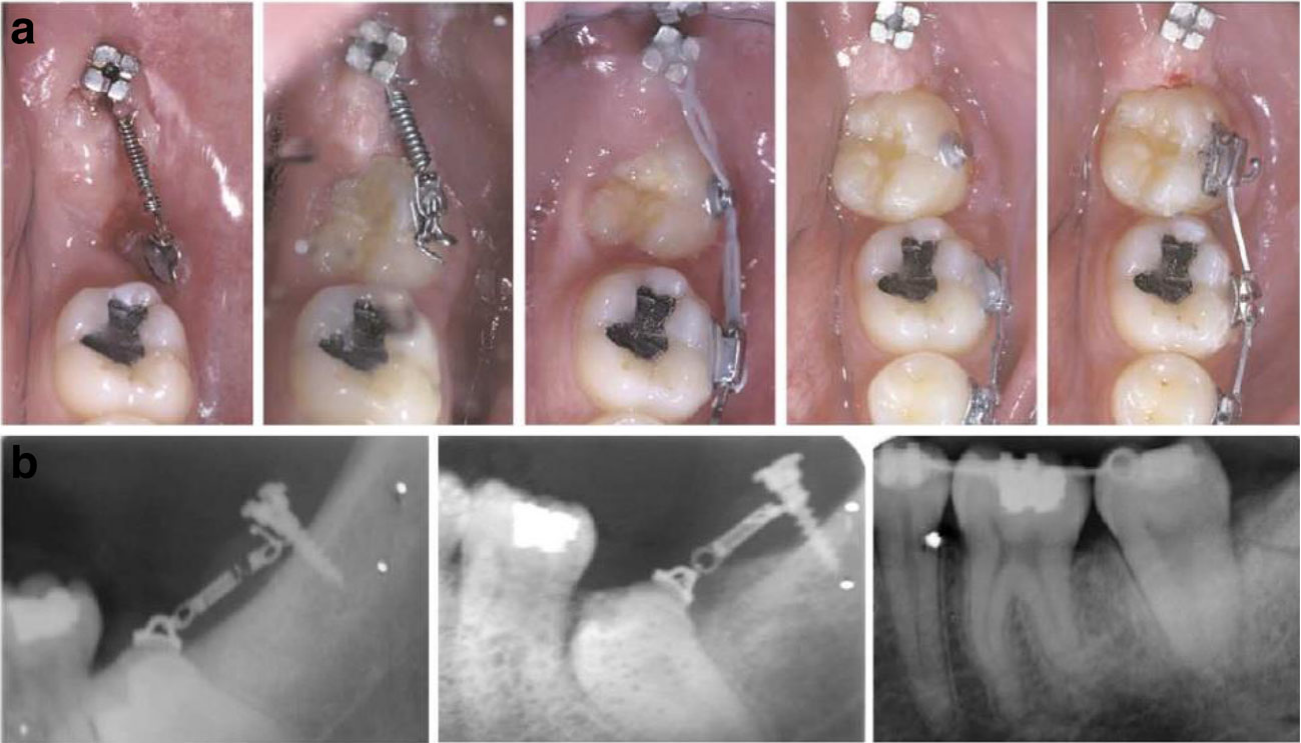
Uprighting of an impacted #37 with miniscrew implant and a closed coil spring tied to an orthodontic bracket. a A 7-mm titanium miniscrew inserted in the retromolar area and loaded with 50-g force on nickel-titanium closed coil spring. After the extrusion of the crown, the tooth was uprighted with fixed sectional appliance. b Progress radiographs during the treatment (from Giancotti et al. [3], with kind permission of American Journal of Orthodontics and Dentofacial Orthopedics)
